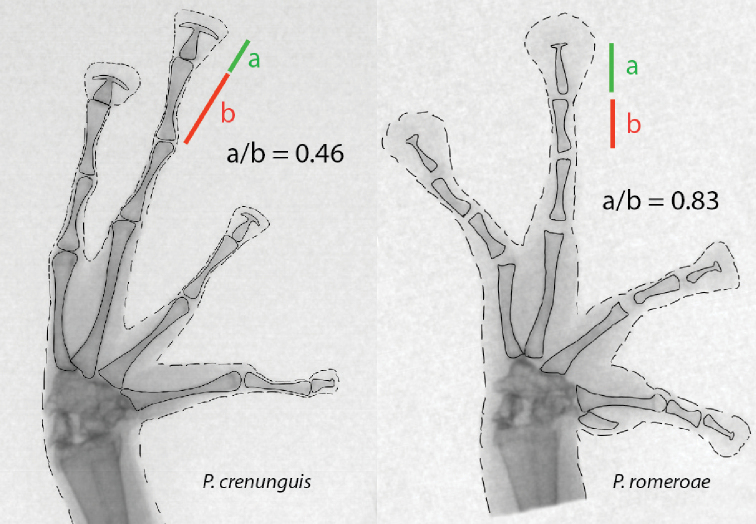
X-ray images of the left hand of adult *Pristimantis
